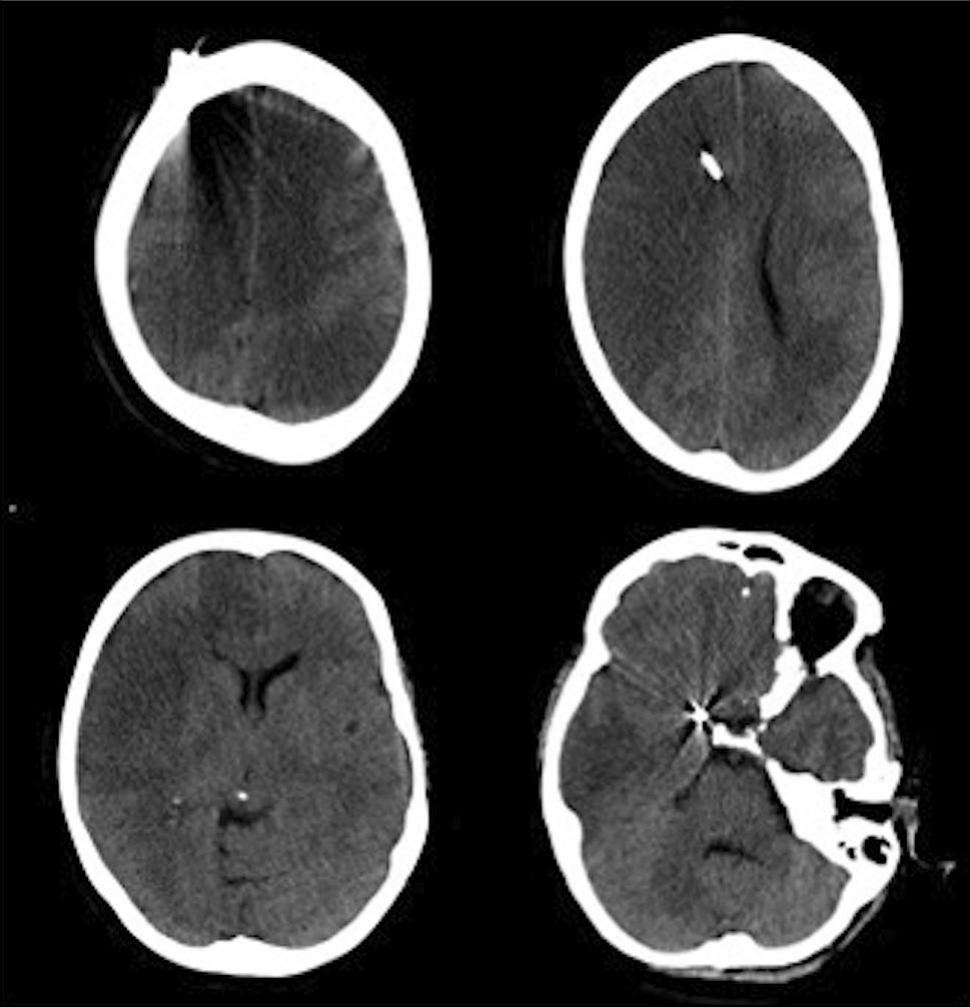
CT scan of a patient with low grade aSAH (in this case HH 1) and cerebral infarct unrelated to treatment
